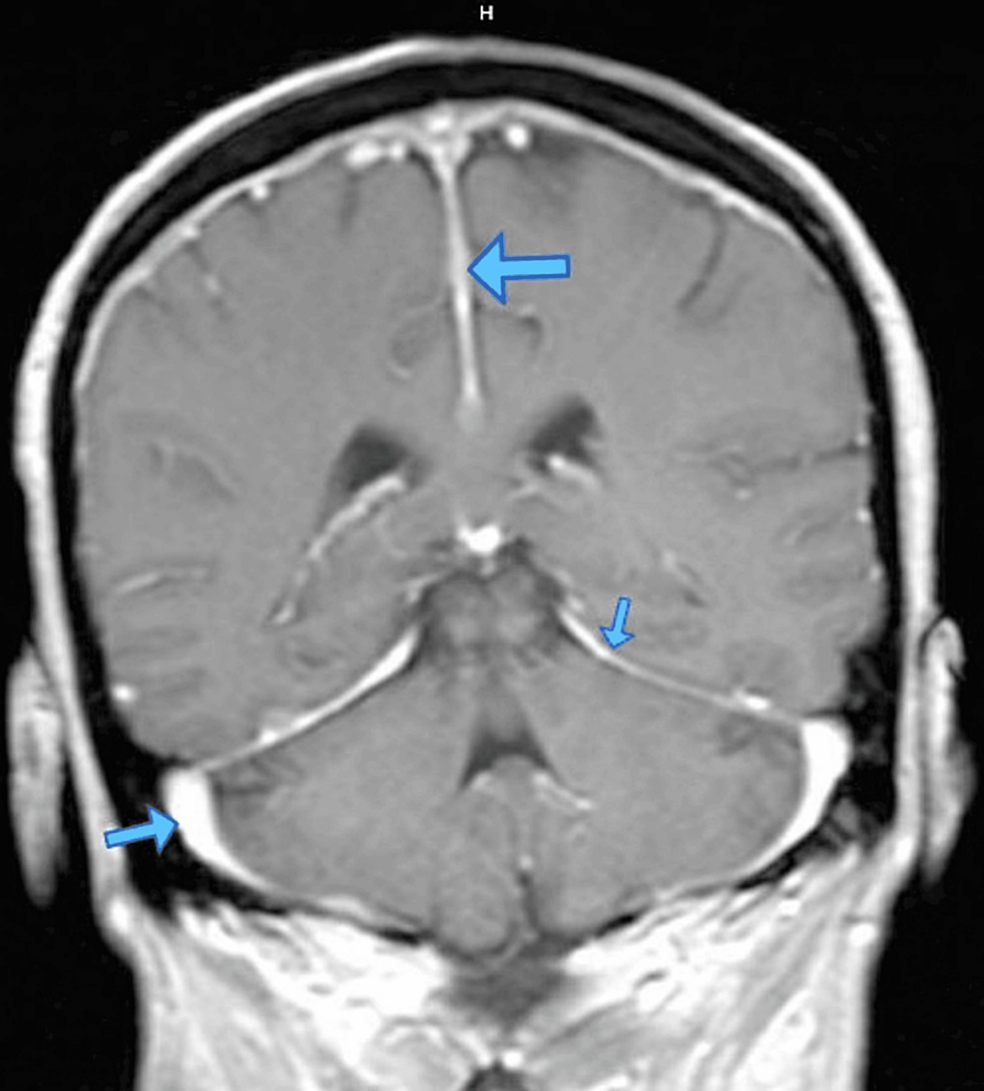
MRI of brain w/wo gadolinium contrast, T1 coronal view Blue arrows pointing toward enhancement of the meninges
